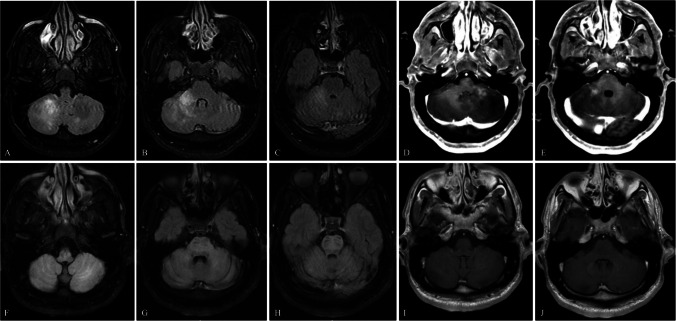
A-E: MRI brain with and without contrast at time of PML symptom onset (October 2024). Images A-C show axial T2/FLAIR signal abnormalities. Images D, E show axial T1 post-contrast enhancement. G-J: MRI brain with and without contrast at time of PML symptom stability (April 2025). Images F–H show axial T2/Flair signal abnormalities. Images D, E show axial T1 post-contrast enhancement resolution
